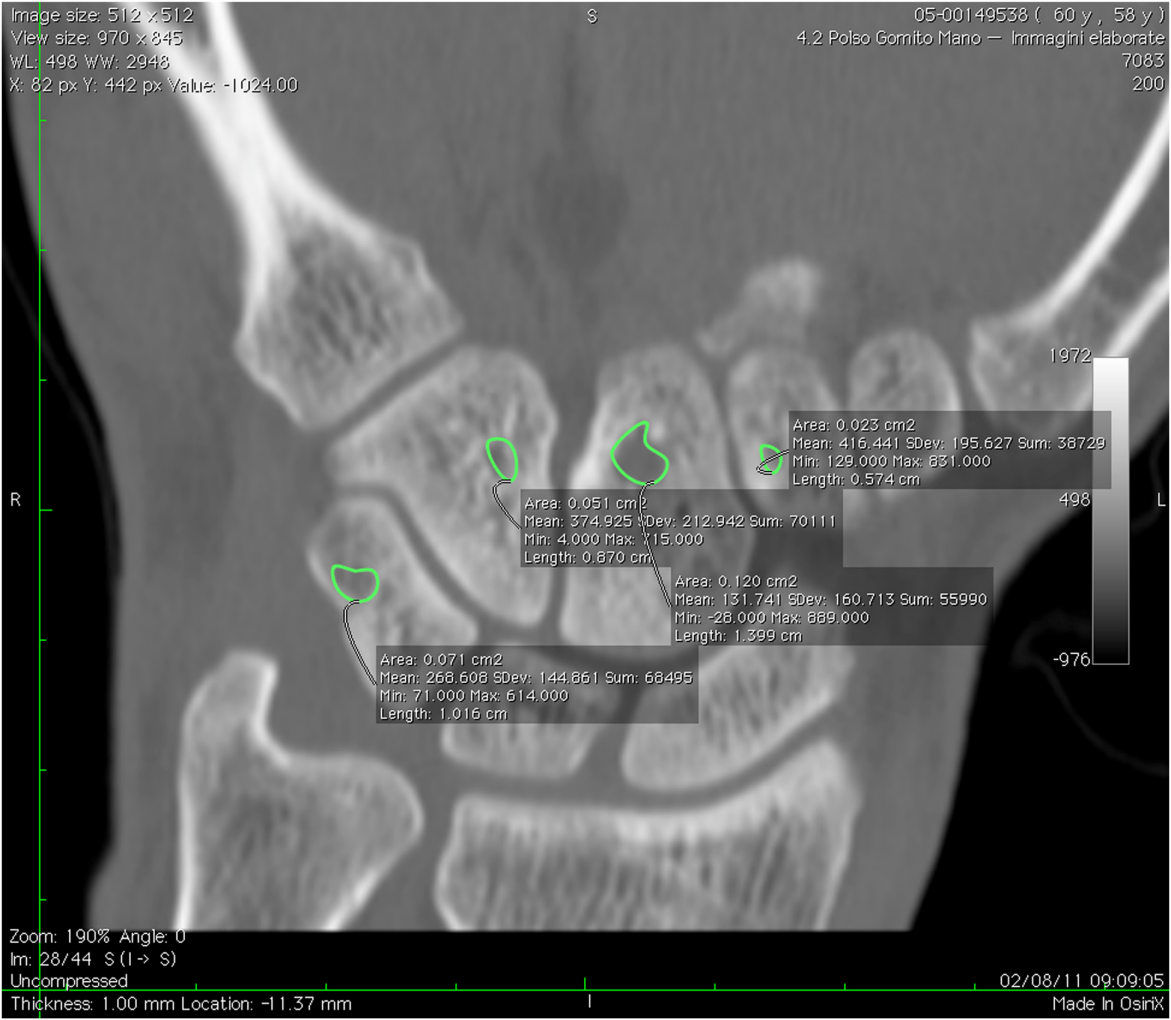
CT coronal reconstructions of wrist. Magnification of erosions of triquetrum, hamate, capitate and trapezoid. Each area was automatically obtained by defining the contour of the erosion.
